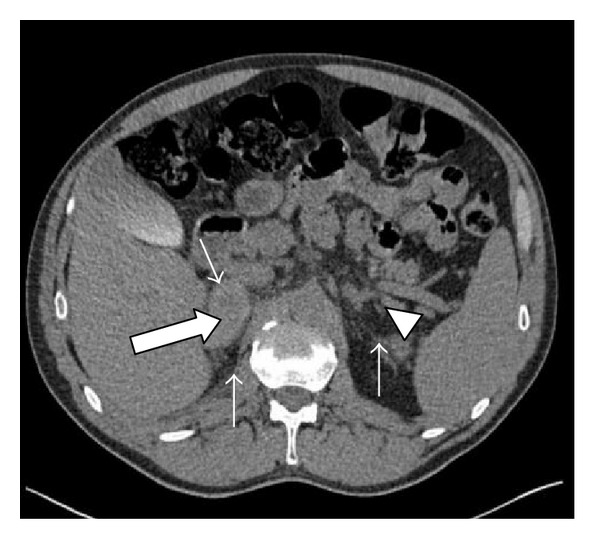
Adrenal CT without contrast reveals right adrenal mass (thick arrow) and thickening of the left adrenal gland (arrow head). Soft tissue stranding is indicated by the thin arrows.
